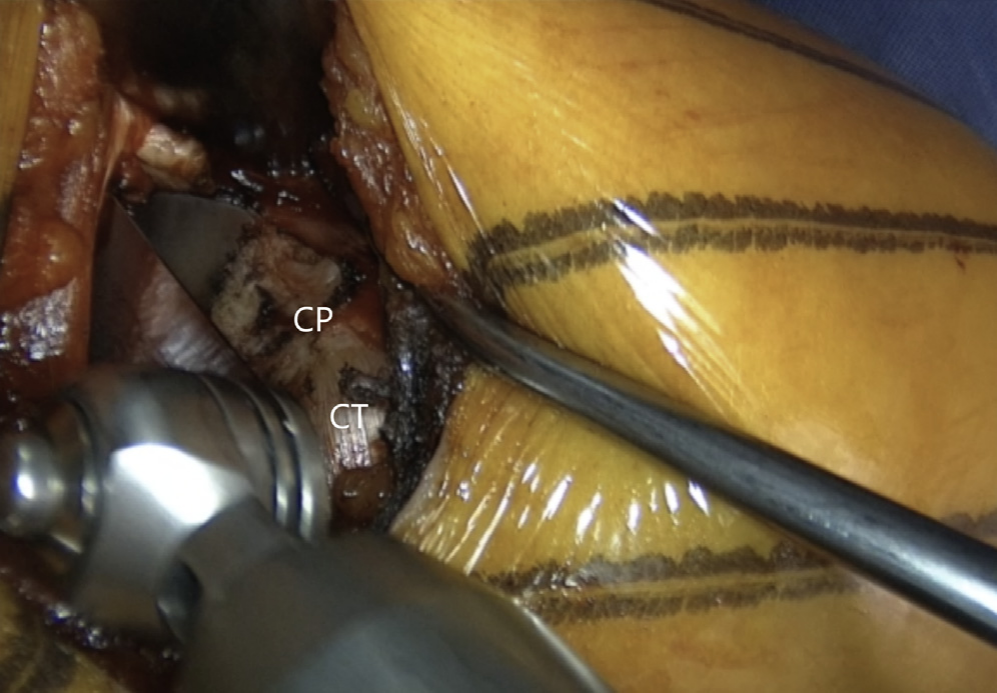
Left shoulder of a patient placed in a semi–beach-chair position. A 90° angled saw blade is used for coracoid osteotomy, which is performed at the base of the coracoid process (CP) as far back as possible, however, still in front of the coracoclavicular ligaments. (CT, conjoined tendon.)
